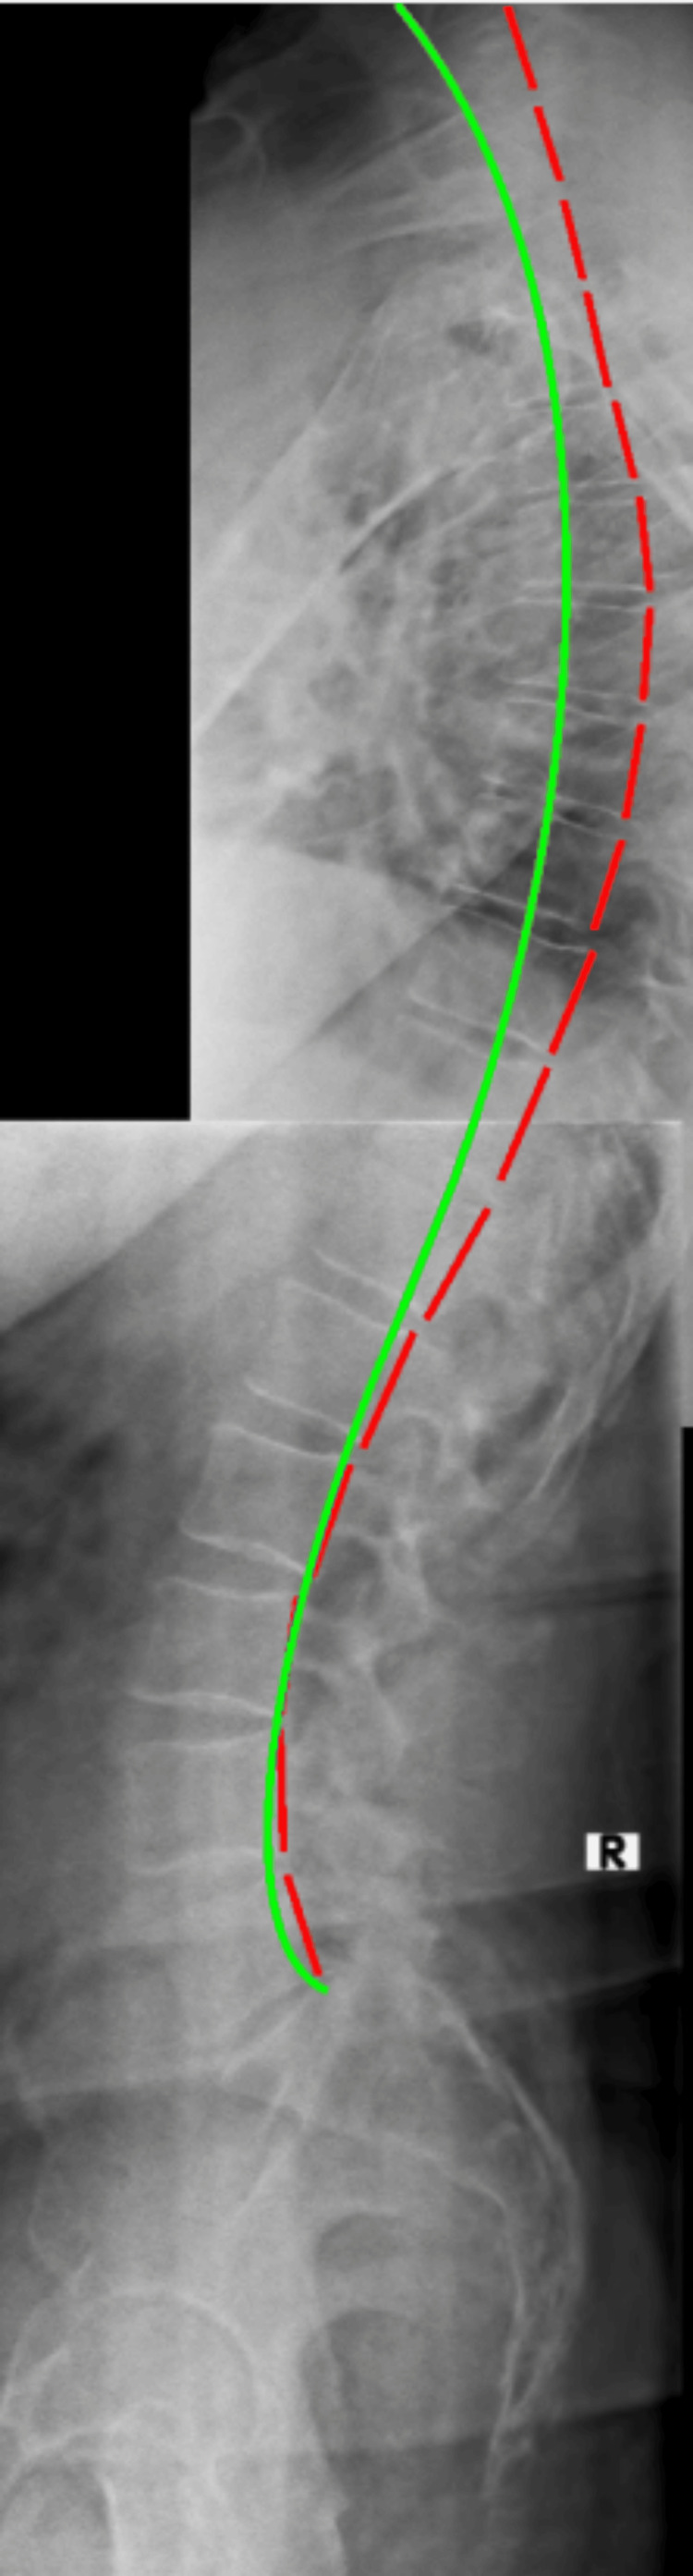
Initial lateral thoracic and lateral lumbar radiographs stitched for sagittal balance analysis The red dashed line measures the posterior tangents of the vertebral bodies using the Harrison Posterior Tangent Method. The green line represents ideal thoracic kyphosis and lumbar lordosis.
